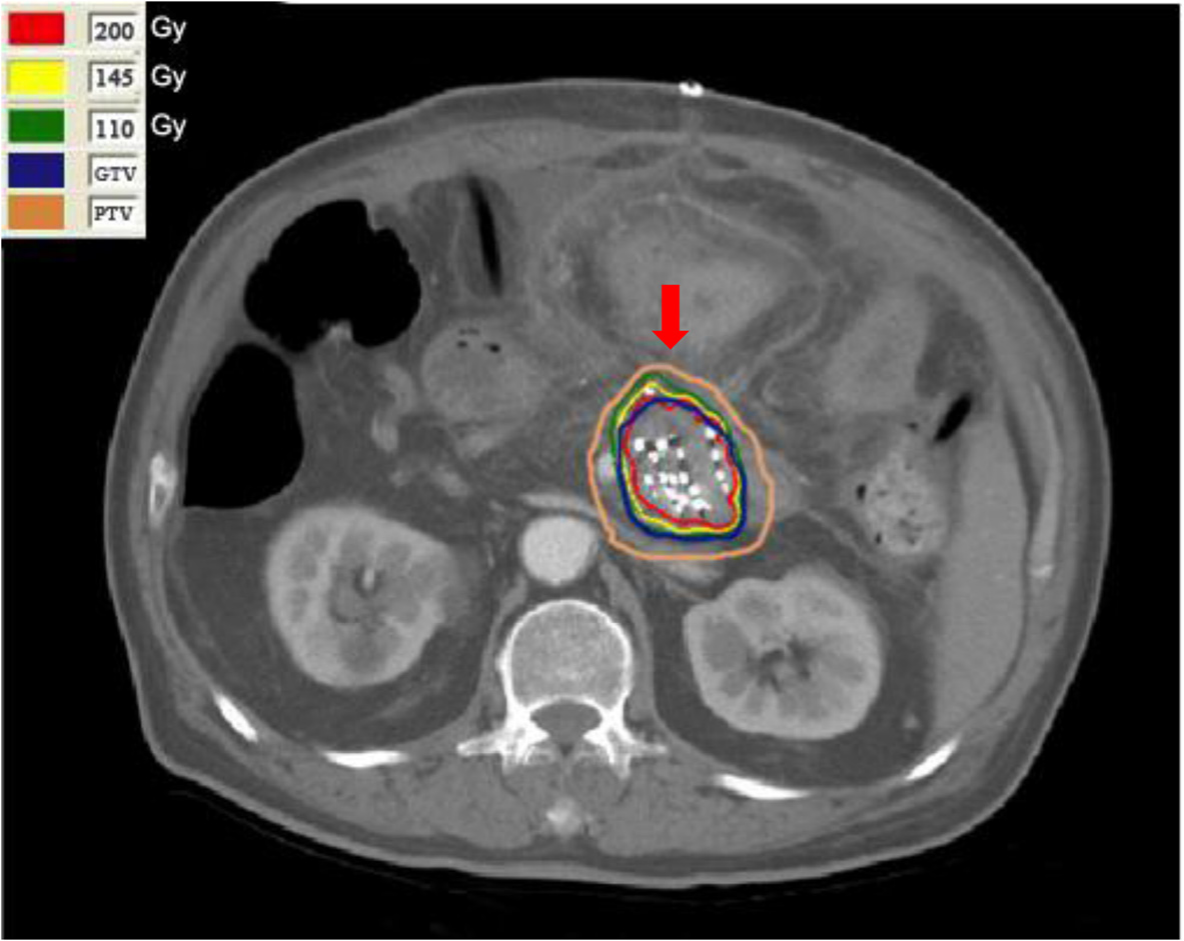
CT image and dose distribution curves of a typical patient. Male, 63 years old, stage III, T4N0M0. The green line is the isodose curve for 110 Gy.
